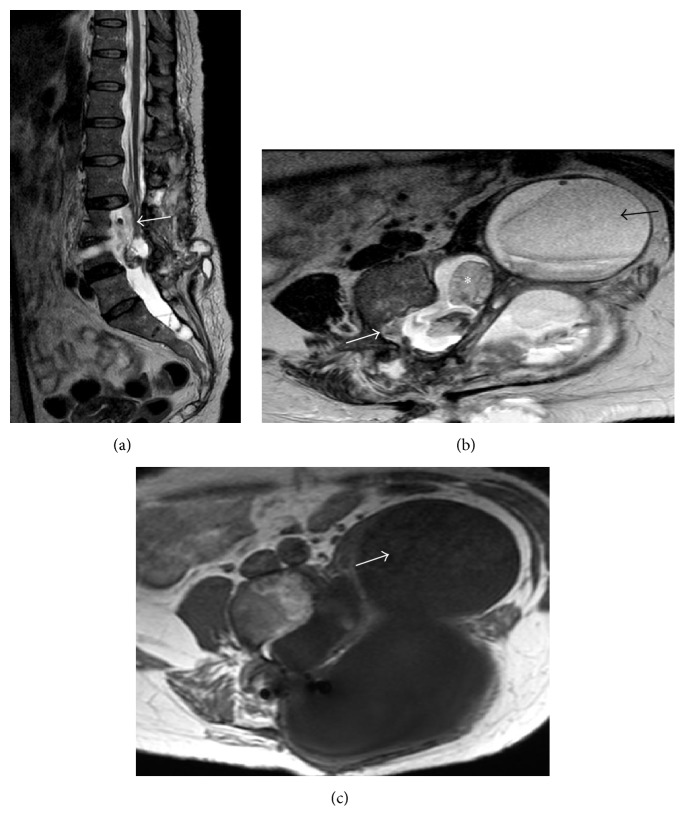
Sagittal and axial T2 weighted (a and b) and axial T1 weighted postgadolinium MRI images at six months after the third operation showed a new giant pseudomeningocele with intra-abdominal extension. The sagittal and axial T2 weighted MRI images (a and b) demonstrated dural sac and cauda roots compression (white arrows) and the inhomogeneous non-CSF like content of the pseudomeningocele (black arrow) probably due to the presence of surgical patch materials and blood products. Axial T1 weighted after gadolinium MRI picture (c) excluded pathological enhancement (white arrow).
